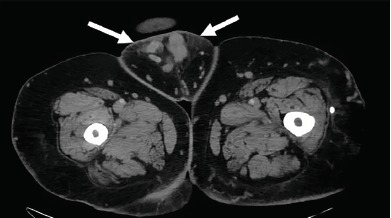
Axial view of computed tomography of abdomen and pelvis with intravenous contrast demonstrating fat stranding (arrows) caused by inflammation from thrombophlebitis.
